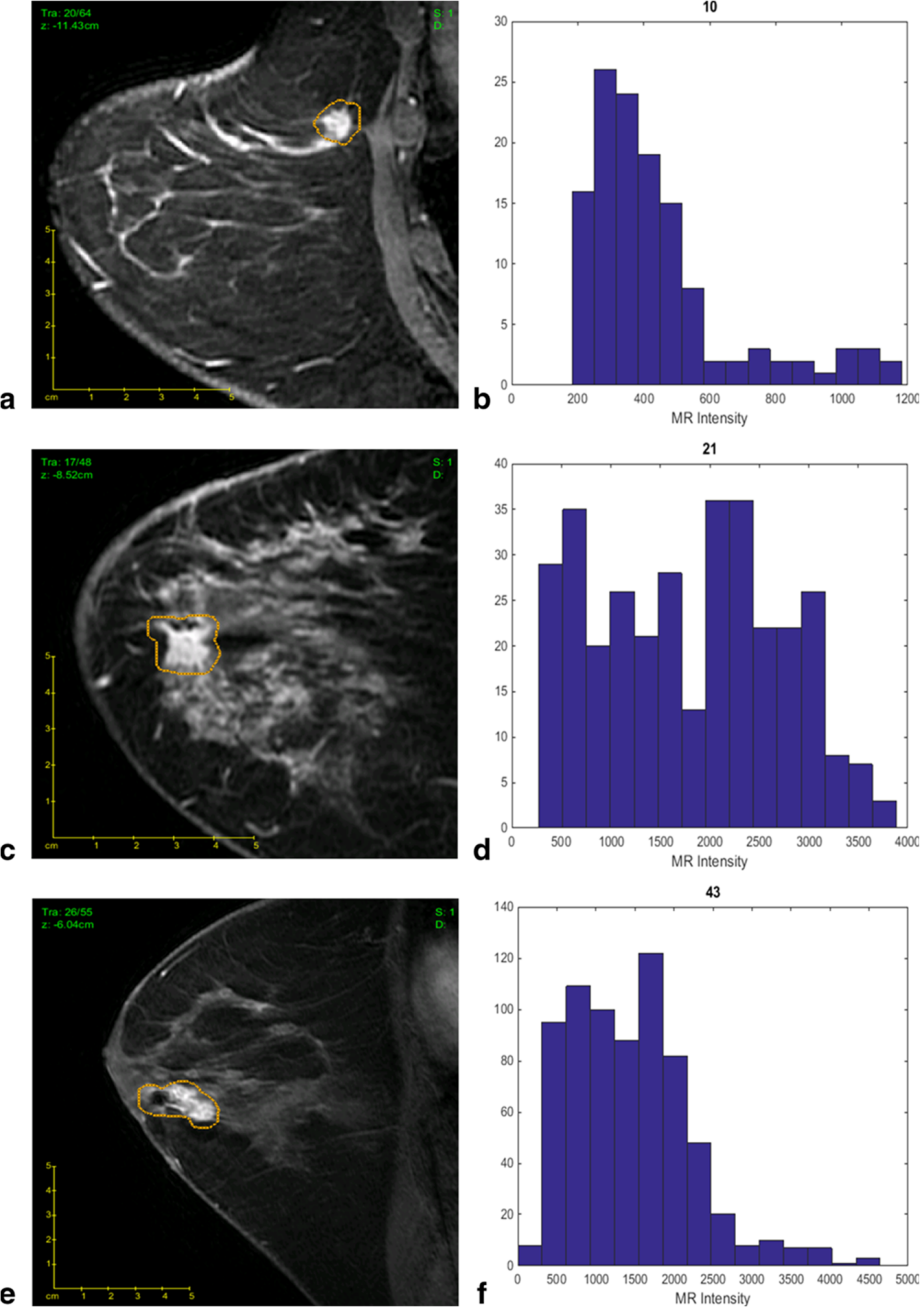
The best-fit linear regression model allows imaging features to differentiate tumors with different Oncotype Dx Recurrence Score (ODxRS). a Sagittal T1-weighted fat-suppressed post-contrast MRI of an invasive ductal nuclear grade 1 carcinoma with an ODxRS of 10 and (b) corresponding kurtosis histogram, which demonstrates the frequency of MR intensity. c Sagittal T1-weighted fat-suppressed postcontrast MRI of an invasive ductal nuclear grade 2 carcinoma with an ODxRS of 21 and (d) corresponding kurtosis histogram. e Sagittal T1-weighted fat-suppressed postcontrast MRI of an invasive ductal nuclear grade 3 carcinoma with an ODxRS of 43 and (f) corresponding kurtosis histogram. Reprinted with permission from: J Magn Reson Imaging. 2015 Nov;42 [5]:1398–406. doi: 10.1002/jmri.24890 Breast cancer subtype intertumor heterogeneity: MRI-based features predict results of a genomic assay. Sutton EJ, Oh JH, Dashevsky BZ, Veeraraghavan H, Apte AP, Thakur SB, Deasy JO, Morris EA.
